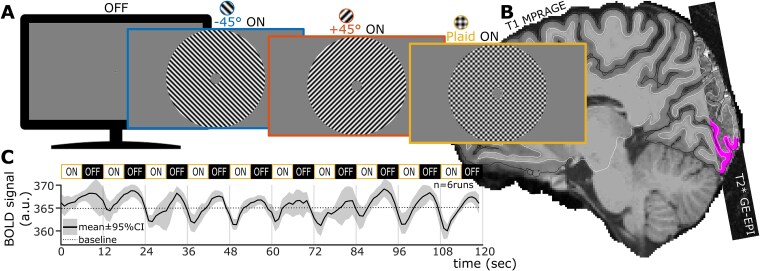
Experiment overview. A) Stimuli presented during OFF (fixation only) and ON (fixation + contrast-reversing grating or plaid overlay) periods. B) Example BOLD functional (T2*-weighted) and anatomical (T1-weighted) images, coregistered using boundary-based registration. Gray matter inner and outer boundaries outlined in dark and white, respectively. Purple overlay shows the V1 gray matter ROI. C) Example BOLD timeseries averaged across the ROI (no spatial feature selection applied) and across runs of the plaid stimulus condition. Data from participant 03sk. Confidence intervals derived from 8,192 bootstrap resamples with replacement.
